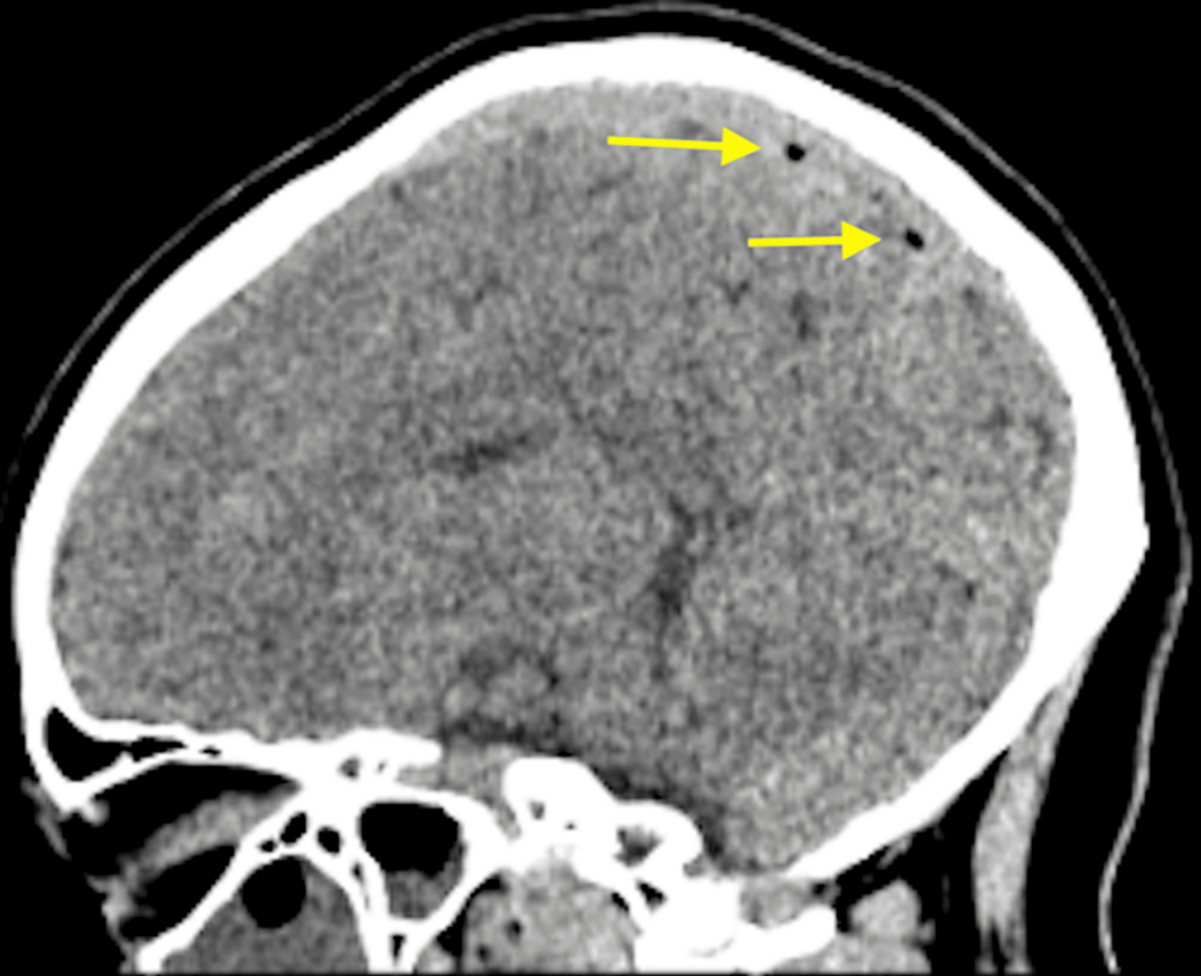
Unenhanced sagittal computed tomography image of the brain demonstrates air present within the superior sagittal sinus.
